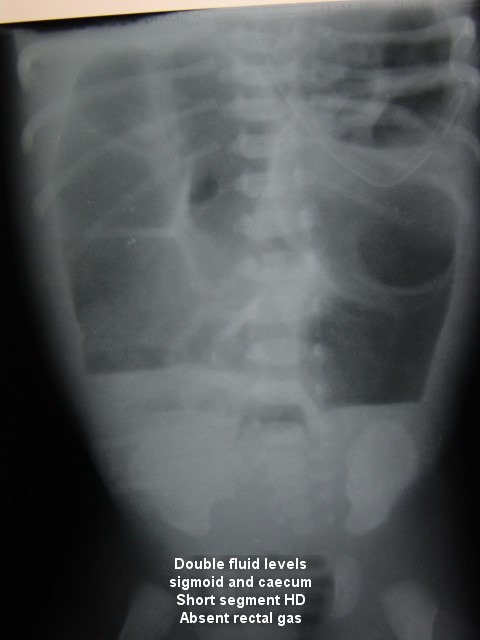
Figure 3: X-ray showing absent gas in the pelvis- aganglionosis of distal colon.
